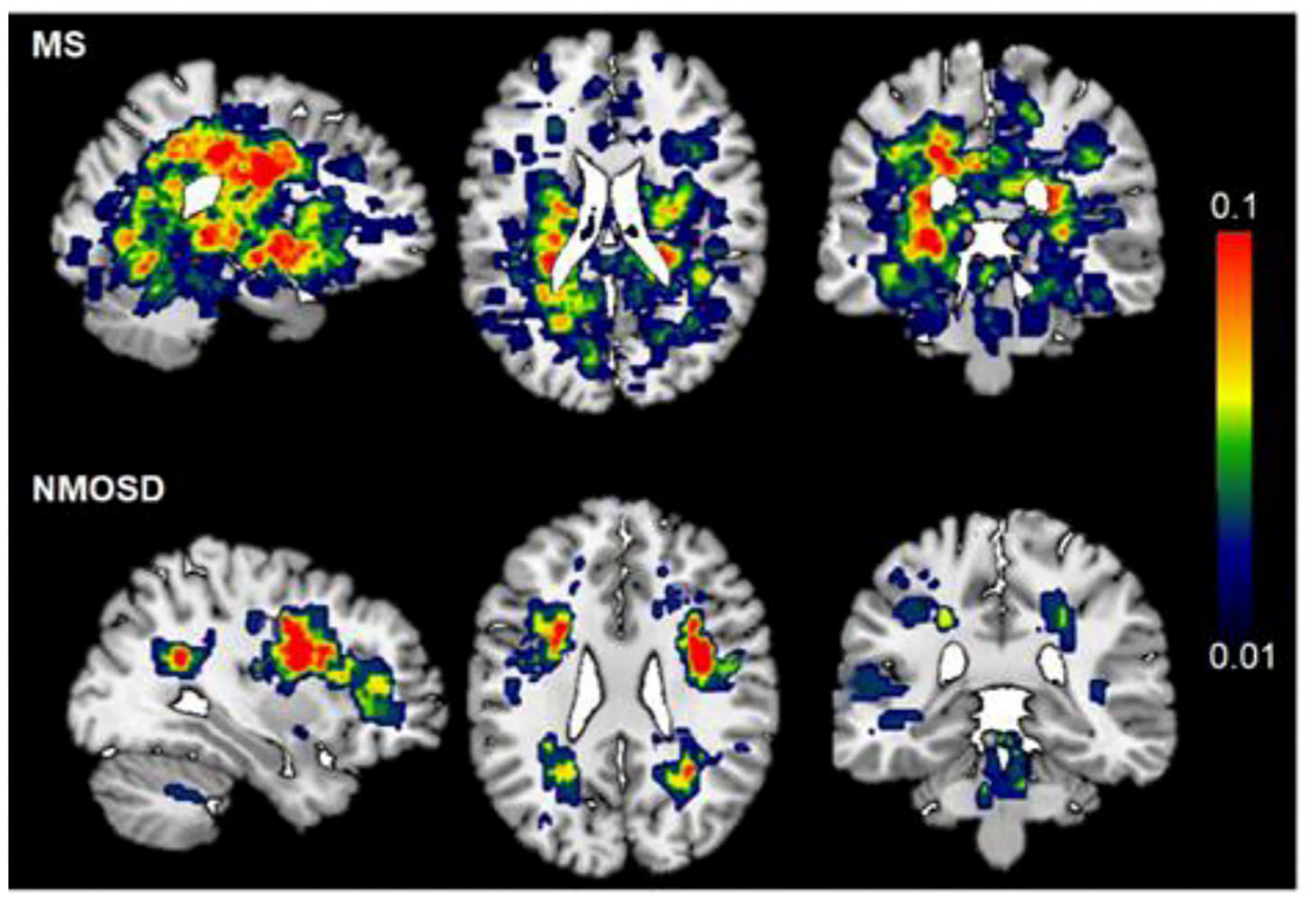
Probability map of brain lesions in patients with NMOSD and MS. T2-weighted lesion probability maps in MS (upper, *n* = 85) and NMOSD (lower panel, *n* = 90) patients. The lesion mask of each patient was created on top of the Montreal Neurological Institute standard 2 mm T1WI template brain (MNI152). The color bar denotes the probability range (1–10%). MS has more brain lesions than NMOSD. The highest probability of brain lesion distribution was periventricular in MS patients, deep white matter in NMOSD.
